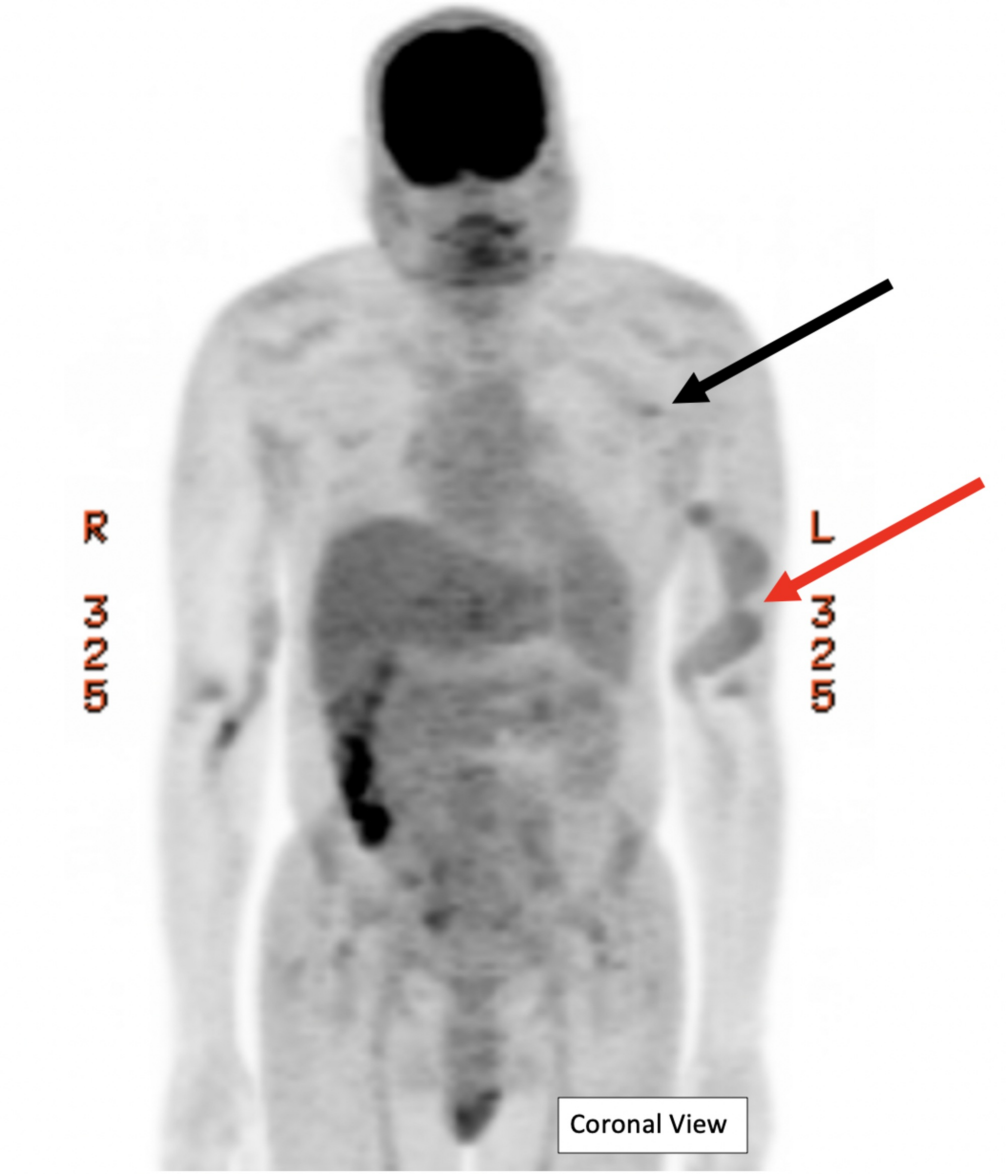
F-18-Fuorodeoxyglucose Positron Emission Tomography (FDG PET) Scan A diagnostic FDG PET scan found that the left upper extremity fistula had mild FDG uptake activity (red arrow) with standard uptake value (SUV) in the range of 1.7-2.1. The catheter extending to the left axilla (black arrow) shows moderate abnormal activity distal with SUV 2.1. There was no uptake at the left internal jugular fistula. Right arm fistula has mild activity. Physiologic activity noted in brain, salivary glands, blood pool, liver, and higher activity in colon, cecum, and testes.
